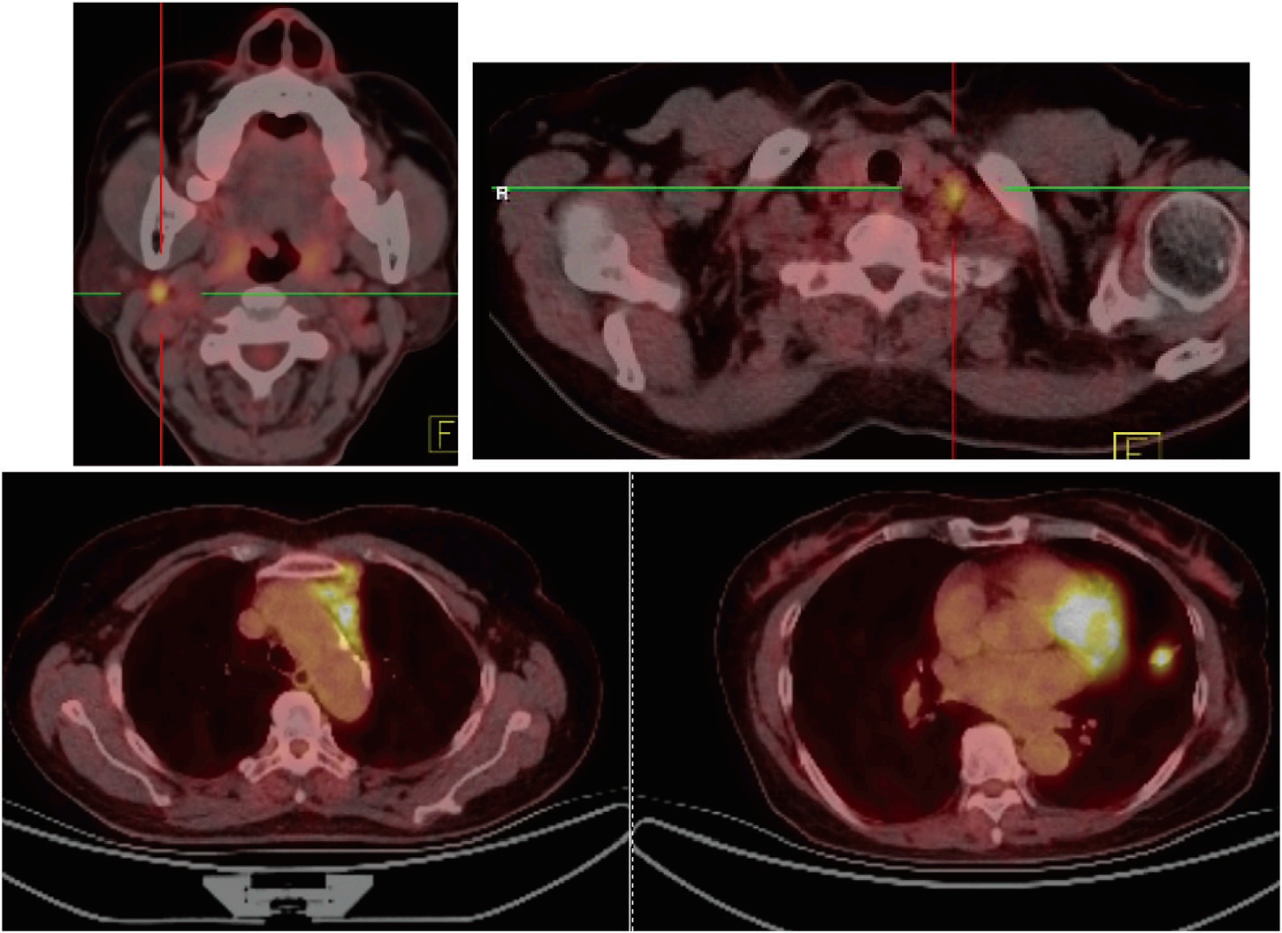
Positron emission tomography/computed tomography imaging. Positron emission tomography/computed tomography imaging on 19 February 2019 revealed an enlarged nodule measuring 1.4 cm × 1.5 cm on let upper lobe (LLL) with a maximum standardized uptake value (SUVmax) of 8.6, right cervical lymph nodes at 0.9 cm × 1.0 cm with SUVmax 8.8.
